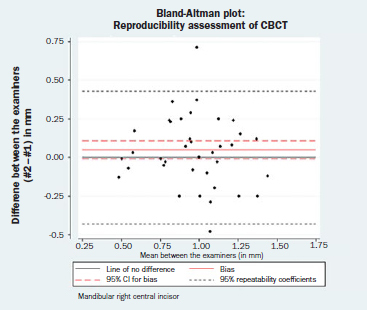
Bland-Altman plot: reproducibility assessment for CBCT measurements, mandibular right central incisor.
